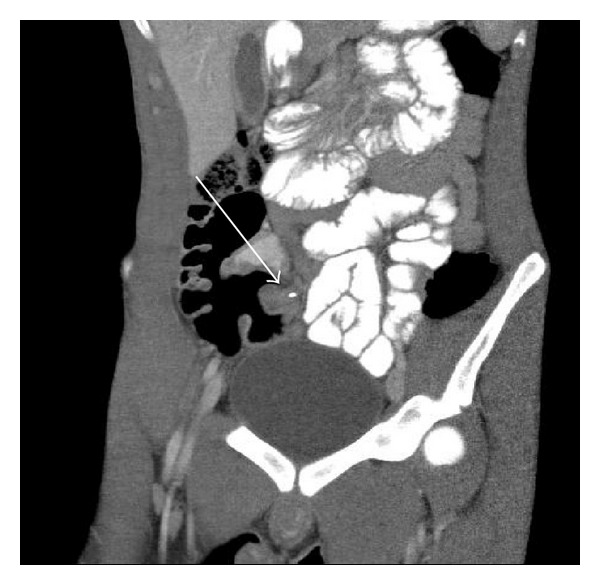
Coronal CT of the abdomen demonstrates dilated, thick-walled appendix (arrow) containing an appendicolith.
